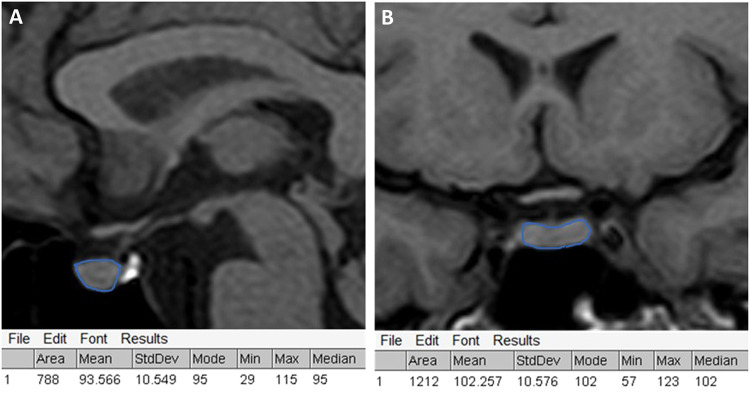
MRI image analysis. Example of marking the region of interest in coronal (A) and sagittal (B) pituitary RM scans and results of the image analysis using ImageJ software.
